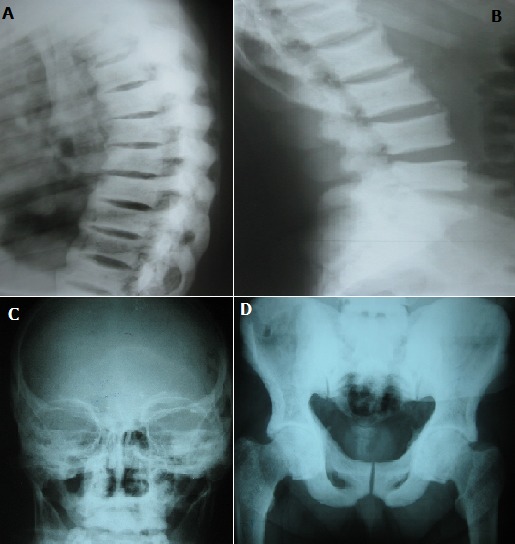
(A) X-ray of the dorsal spine in profile: diffuse bone condensation; (B) X-ray of lumbar spine in profile: diffuse bone condensation; (C)X-ray of the skull: diffuse bone condensation, (D) X-ray of the pelvis: osteosclerosis of the pelvis
